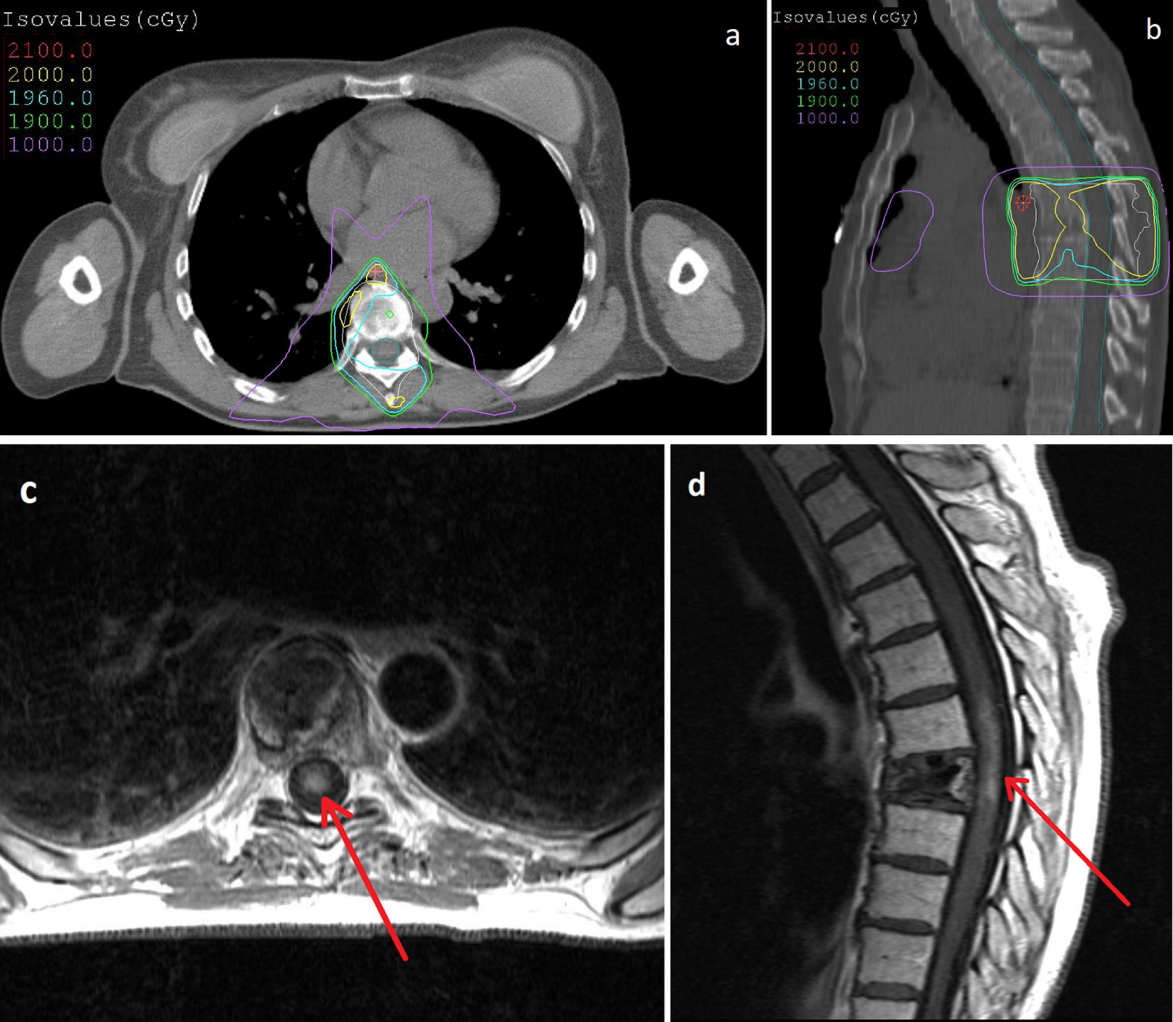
a and b: Treatment planning system (TPS) for T6–T8 irradiation. The balistic (a) was made of 2 right and left posterior oblique beams and one anterior beam. Isovalues lines in axial (a) and sagittal (b) plan showed a homogeneous coverage of the planning target volume (PTV). “Hot spot” (21 Gy) was localised forward rachis (b). Figure 1.c and d: Myelitis on the spinal MRI (T1 SE FAT SAT GADO sequence). After injection of gadolinium chelate, a 36 mm intraspinal contrast enhancement appeared in T6–T7, representing myelitis (d, red arrow). In axial section centred on T6–T7 myelitis predominated in the posterior part of spinal cord (c, red arrow). Cement was visible in T7 vertebral body.
